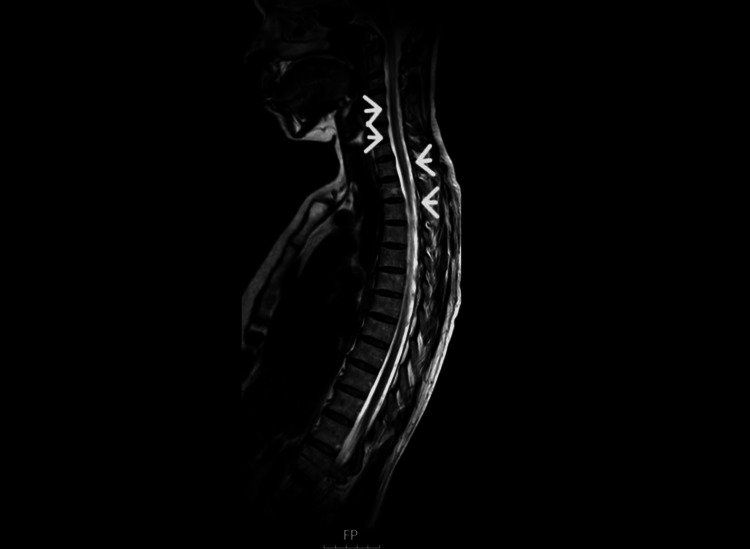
Sagittal view of the TW2 sequence of the MRI spine shows an increased signal in the dorsal and lateral columns of the cervical and thoracic region TW2: Tanner-Whitehouse 2
